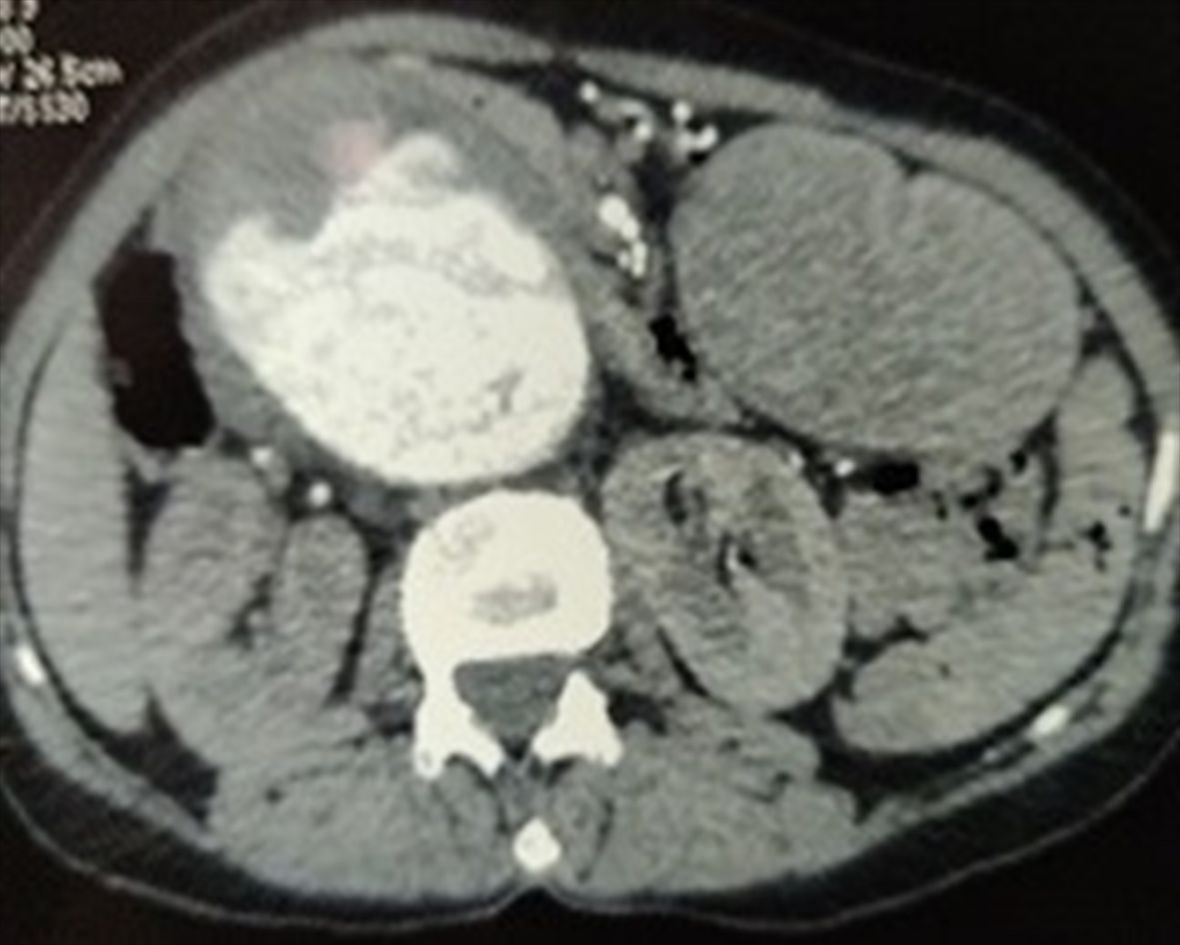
CT angiography of abdominal aorta revealing a large fusiform aneurysm of the abdominal aorta extending from D12 to L5 vertebral bodies, to the bifurcation, with eccentric, irregular, and largely non-calcified thrombus along the right lateral wall.
